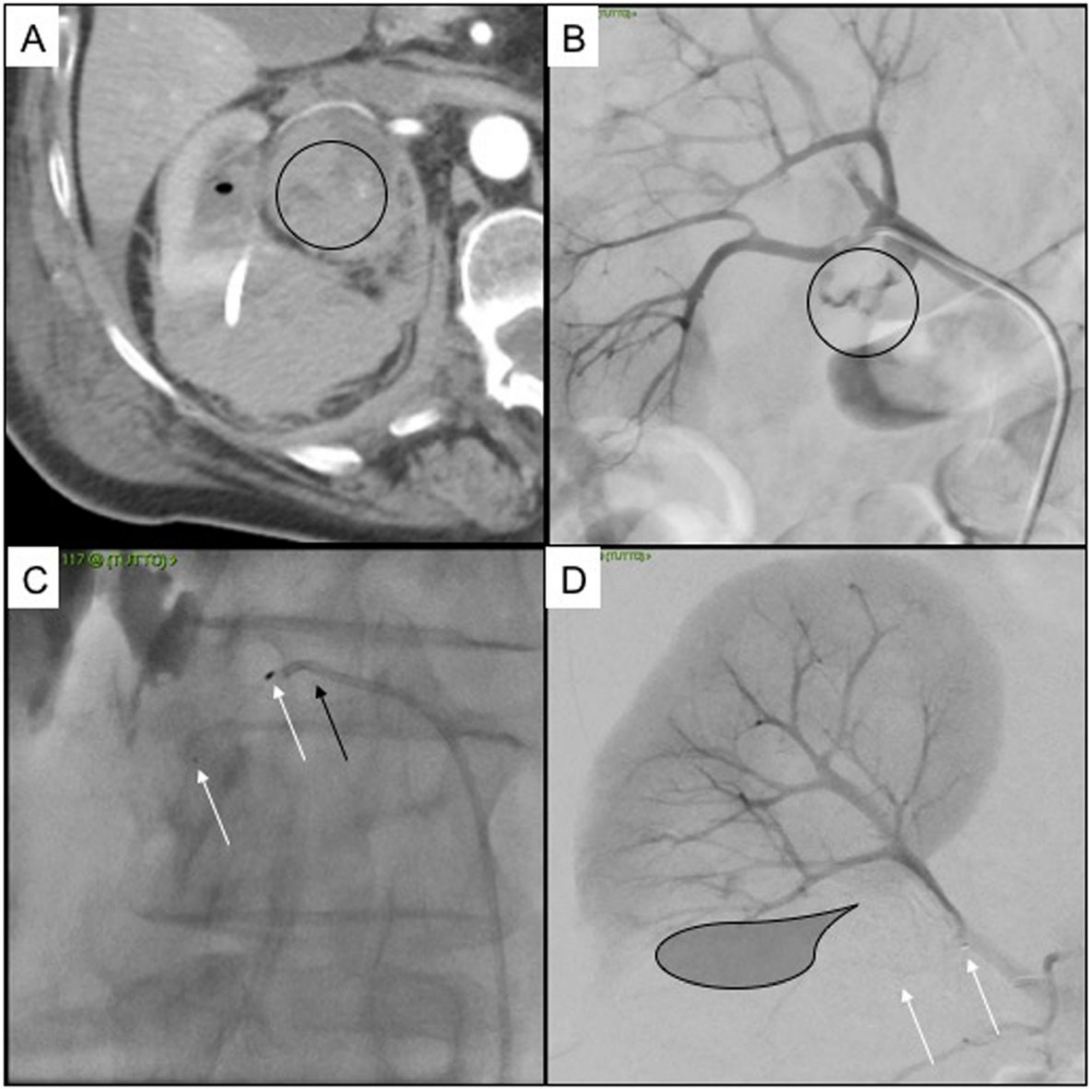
Patient 4. 80 years old female with urinary stones causing right pielectasia, treated with nephrostomy. a Axial CT scan in arterial phase showing active bleeding into the urinary pelvis (black circle), clots are appreciable into the calix (black ovoid), the nephrostomy drainage with perirenal hematoma is appreciable as well; b DSA showing extraluminal blush from the inferior segmental artery (black circle); c selective embolization with MVP 7Q (white arrows indicate distal and proximal radiopaque markers of the MVP, black arrow indicates the 5Fr diagnostic Cobra 1 catheter); d final DSA control showing no more angiographic bleeding (black shadow indicates the ischemic area, white arrows indicate distal and proximal radiopaque markers of the MVP)
